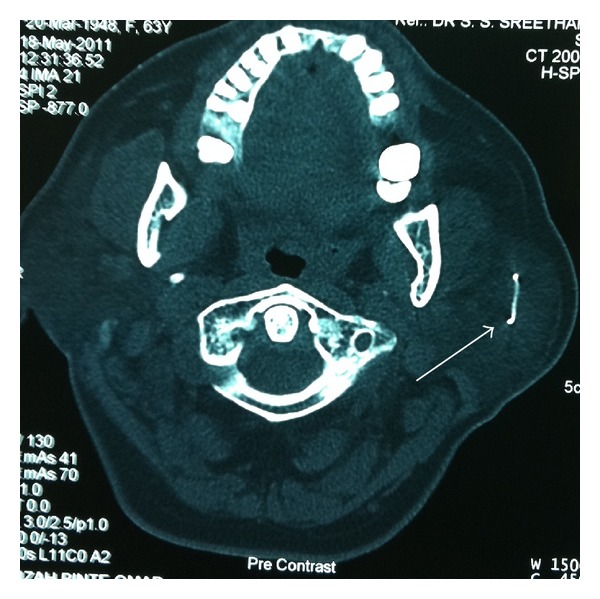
CT scan showing a linear calcification in the substance of the parotid gland (arrow).
